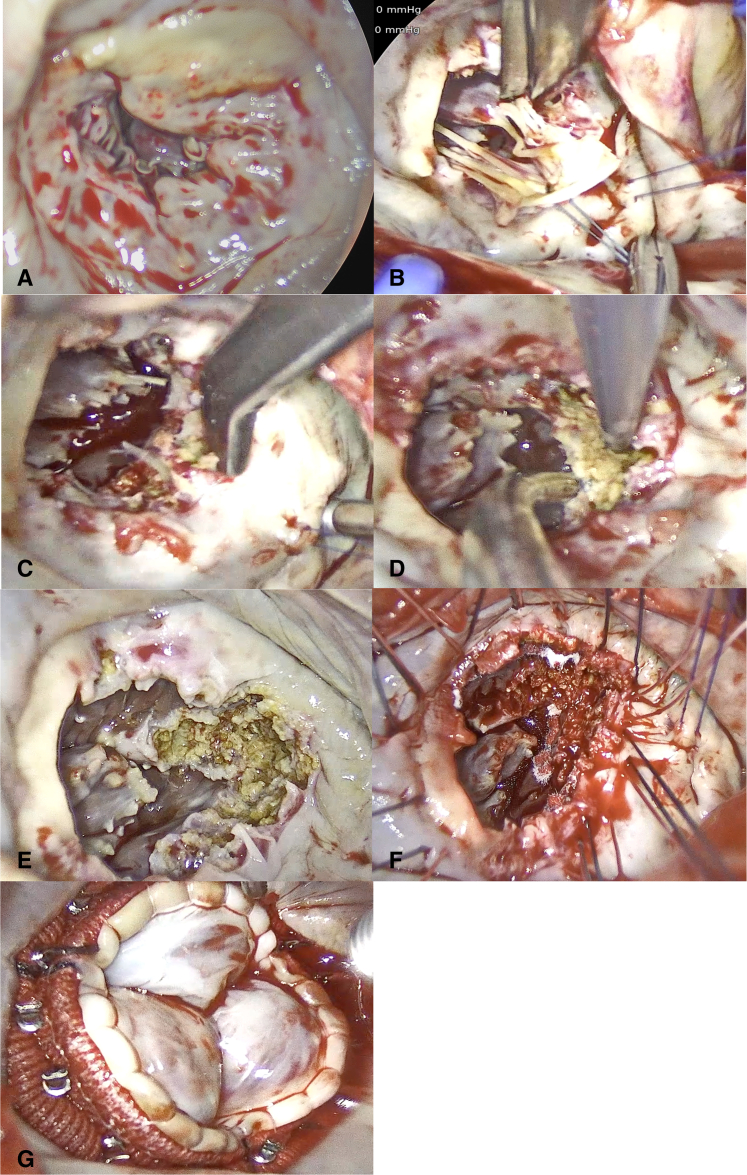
Key surgical steps. A, Mitral valve (*MV*) assessment. B, Anterior mitral leaflet resection. C, Manual debridement. D, Cavitronic ultrasonic surgical aspirator (*CUSA*) decalcification. E, Final result following manual debridement and CUSA decalcification. F, Placement of noninverting pledgeted mitral annular sutures. G, Seated bioprosthetic MV.
